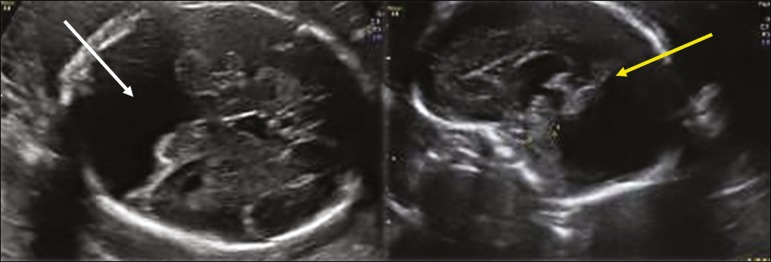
Axial ultrasound of a Dandy-Walker malformation, showing communication between the fourth ventricle and the cisterna magna (white arrow). Sagittal ultrasound showing an abnormal fastigium, vermian agenesis/hypoplasia with upward rotation, and elevation of the tentorium (yellow arrow).
